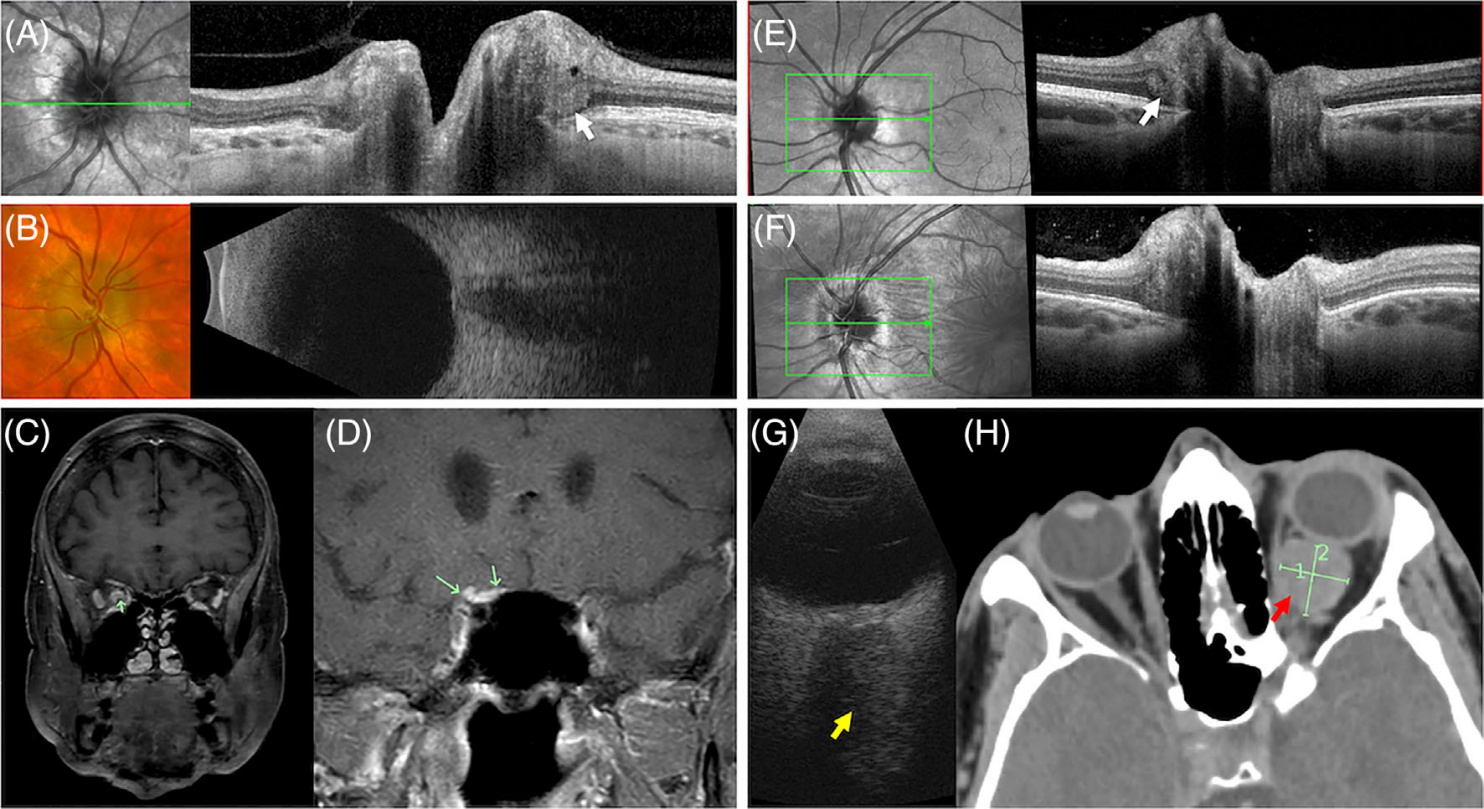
A 66‐year‐old female was diagnosed with optic nerve sheath meningioma 20 years prior when she presented with optic nerve elevation (A‐D). OCT scan through the chronically elevated disc showed PHOMS (white arrow) with intraretinal cystic change at the inner retinal tissue border (A). An elevated optic nerve head was seen on ultrasonography (B). MRI showed circumferential optic nerve sheath thickening and enhancement (green arrow in right orbit, C) with extension of the meningioma posteriorly along the planum sphenoidale attached to a small nodular component (green arrows) along the superior margin of the anterior clinoid process (D). A 30‐year‐old male had a routine optic disc OCT imaging 2.5 years prior to presentation with painful gaze and gaze‐evoked amaurosis (E‐H). The PHOMS that was seen 2.5 years prior (E) but not 11 years prior had enlarged with development of symptoms and new choroidal folds (F). B‐scan ultrasound showed flattening of the posterior globe by an echogenic mass lesion (yellow arrow) adjacent to the optic nerve (G). On computed tomography, the 17 × 17 × 15 mm (markers) intraconal mass (red arrow) that was isodense to the muscle was abutting and flattening the posterior globe and displacing the optic nerve sheath complex inferolaterally (H). Further investigation with magnetic resonance imaging confirmed a low flow vascular malformation.
